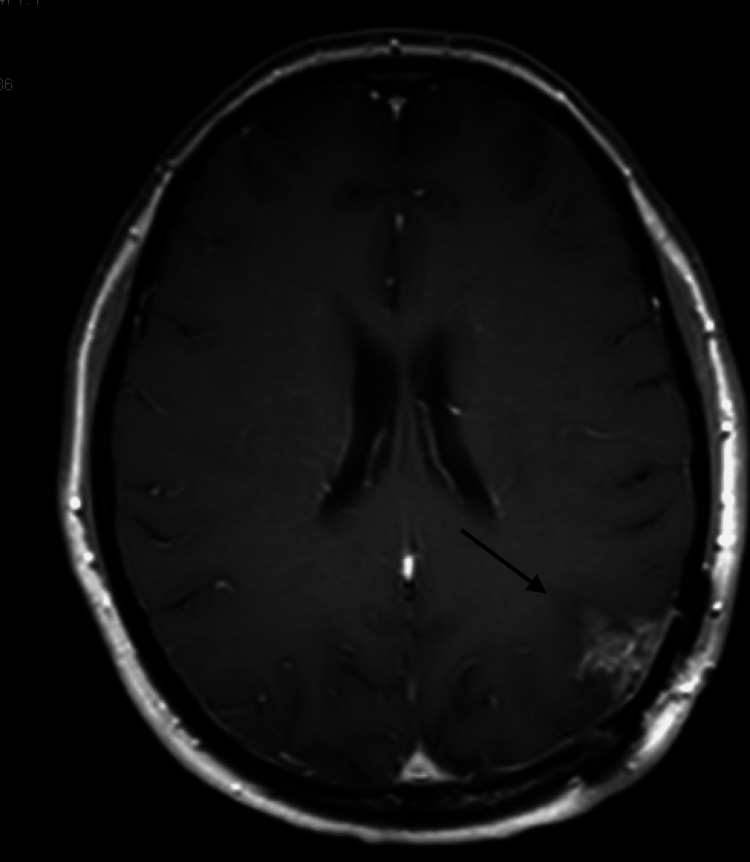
MRI of the brain showing interval development of poorly marginated heterogeneous enhancement of the resection cavity. MRI: magnetic resonance imaging
